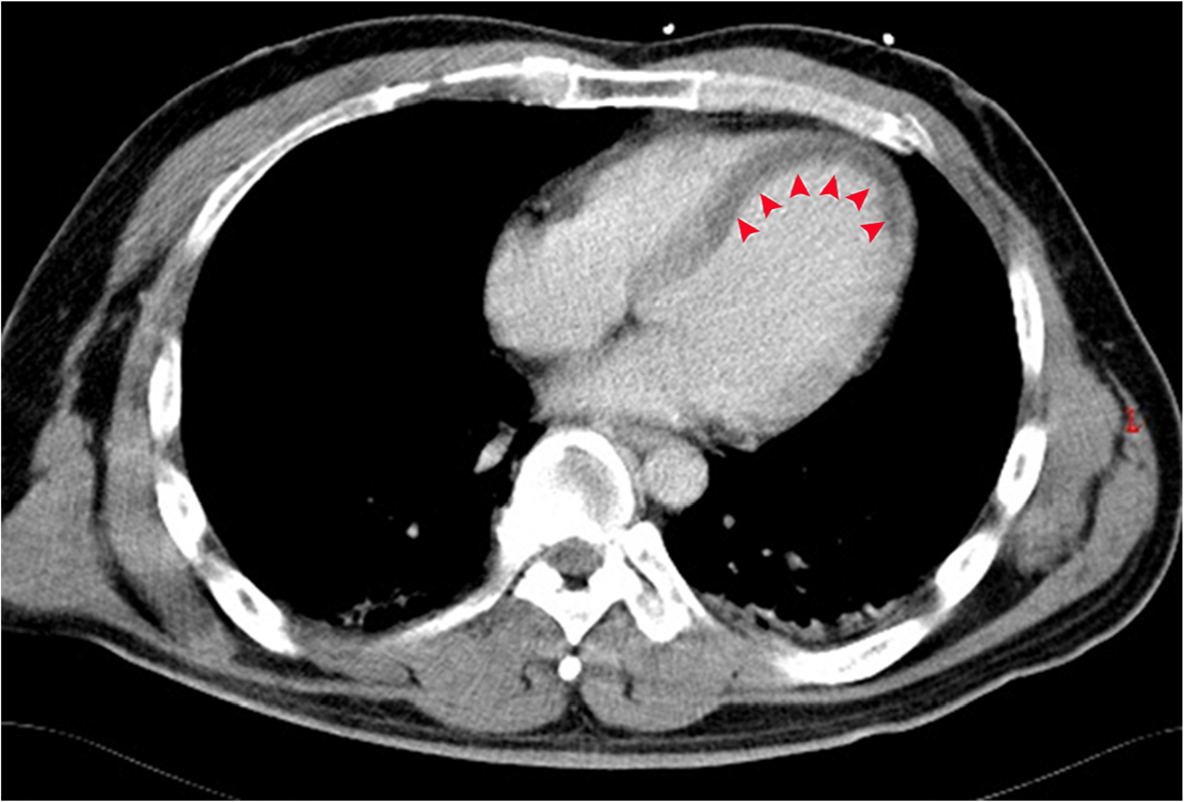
Contrast-enhanced CT revealed decreased myocardial enhancement over the interventricular septum and the left ventricular apex (*arrowhead*)
